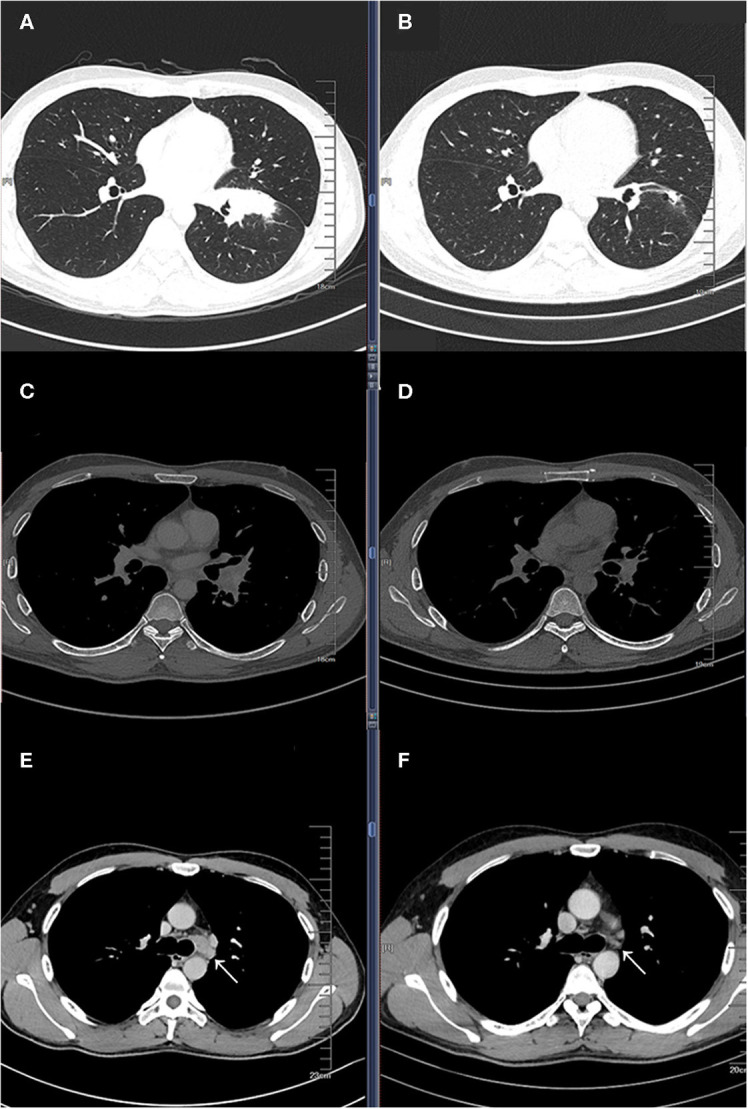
Baseline computed tomography (CT) showing a lung mass in the left lower lobe pulmonary window (A) and mediastinal window (C), and an enlarged left lower paratracheal (4L) lymph node (E); CT images after two cycles of four drug combination therapy showing partial response in the pulmonary window (B) and mediastinal window (D), and a significantly reduction in the 4L lymph node (F).
